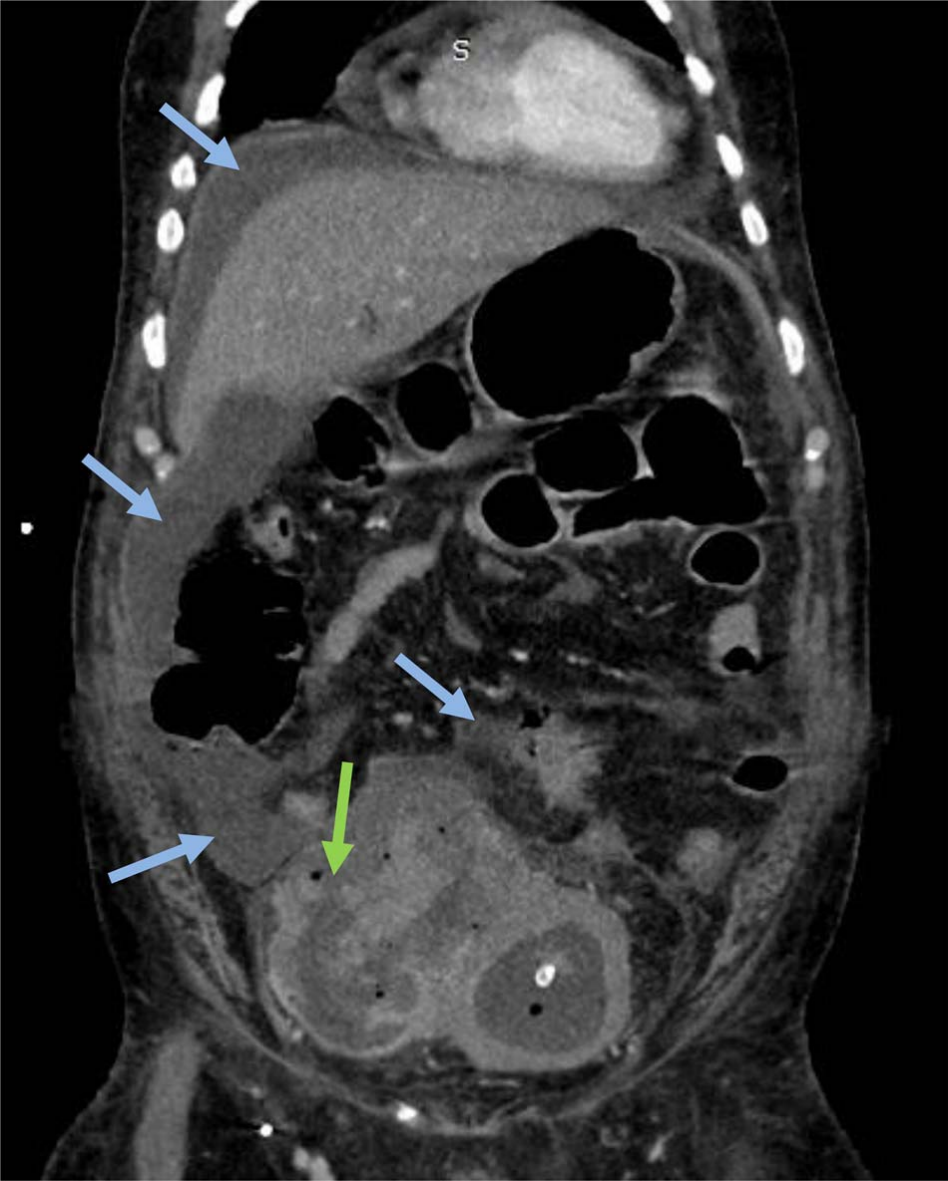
Emergency CT-scan showing intraperitoneal swallowing (blue arrows) due to a fistulization of the bladder (green arrow).
